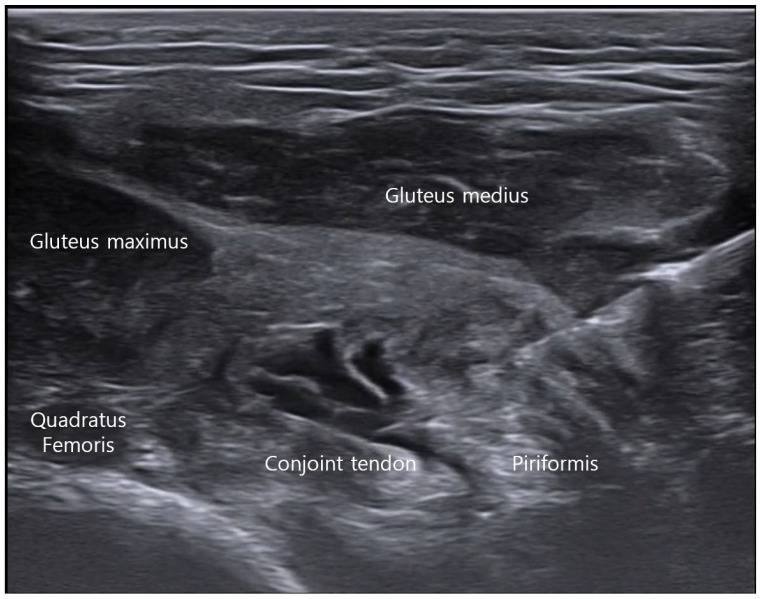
Ultrasound-guided injection into the deep gluteal fascial plane using a cranial-to-caudal in-plane approach. With the cadaver placed in a prone position, the needle was advanced from cranial to caudal using an in-plane technique under real-time ultrasound guidance. The injection was directed into the fascial plane between the short external rotators and the overlying gluteus medius and gluteus maximus muscles.
